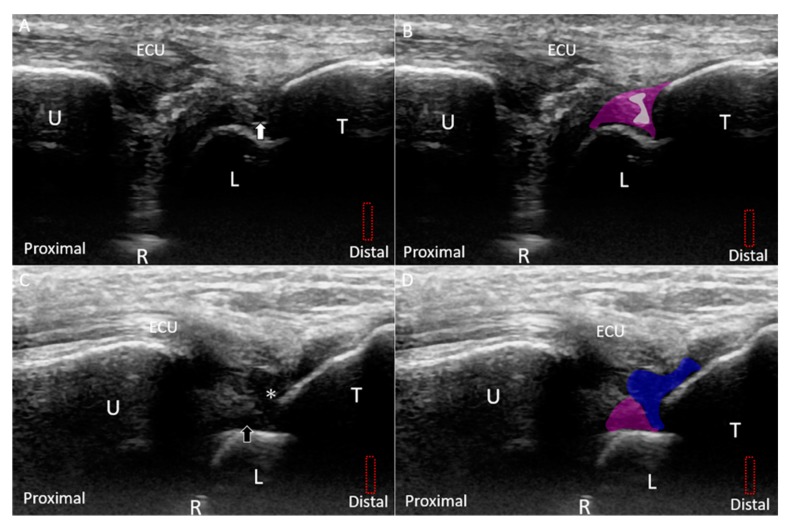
(A) US and (B) superposed US images show a small tear *(white arrow and grey shade)* in the lunotriquetral ligament *(purple shade)* under the long-axis approach. (C) US and (D) superposed US images of the retracted stump *(white asterisk and blue shade)* of the torn lunotriquetral ligament and fluid accumulation *(black arrow and purple shade)*. The different color dashed rectangles are in accordance with the transducer positions in Figure 3. US: ultrasound; U: ulna; R: radius; L: lunate; T: triquetrum; ECU, extensor carpi ulnaris.
